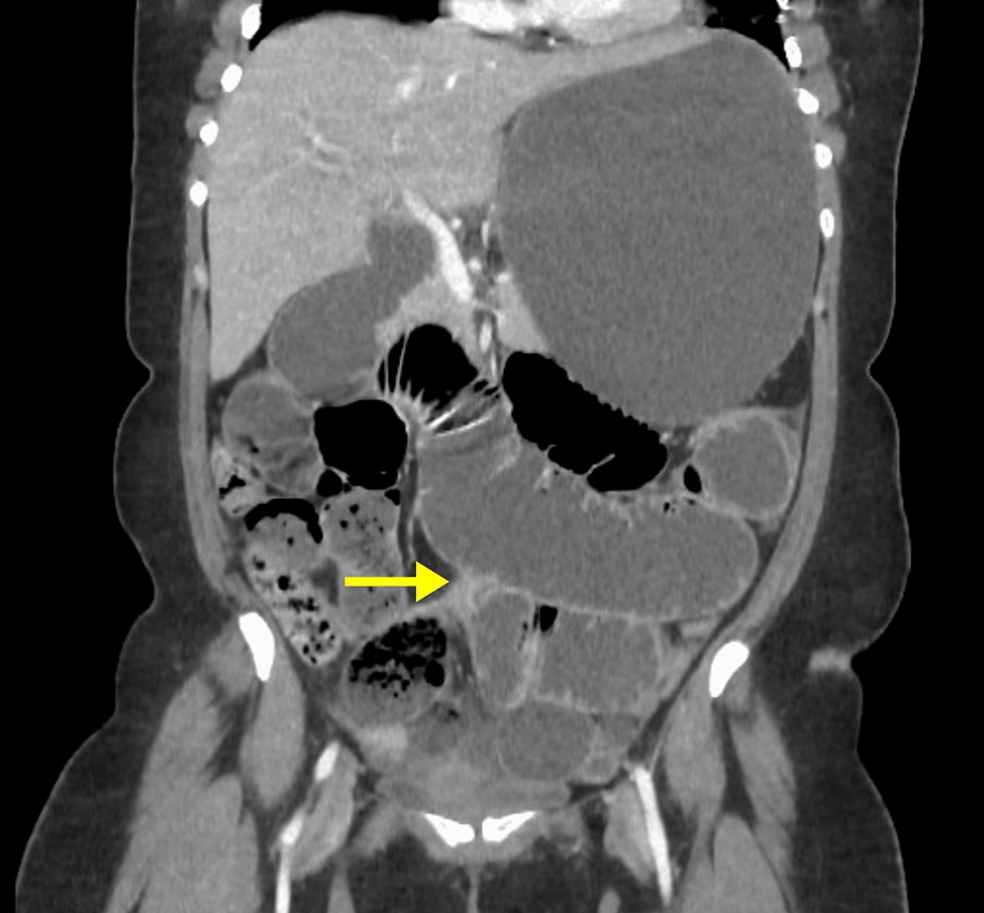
Abdominal CT with contrast shows the small bowel transition zone
